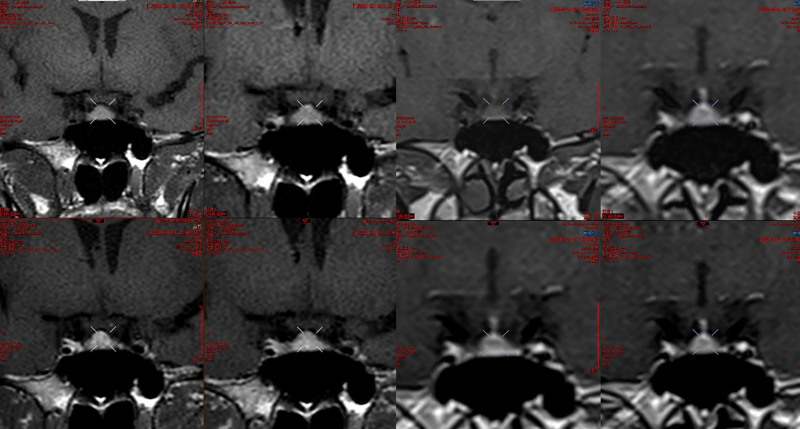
Comparison of pituitary magnetic resonance imaging enhancement between in October 1, 2023, and January 19, 2024.
